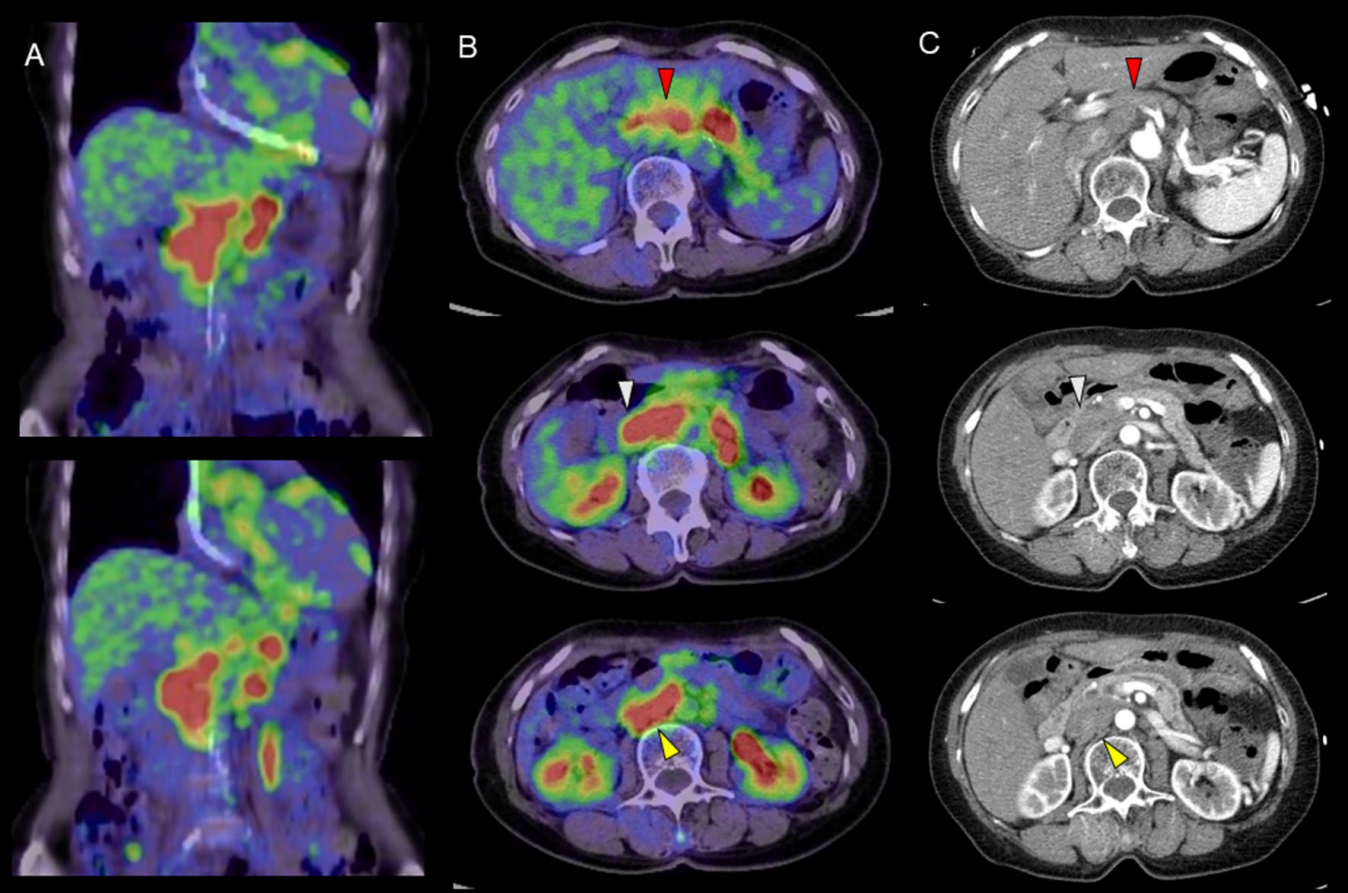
ACoronal images of fused PET/CT image, B axial images of fused PET/CT, C axial images of contrast CT. Intense FDG uptake was observed in multiple swollen lymph nodes: the periportal lymph node (upper row, red arrowheads), anterior pancreaticoduodenal lymph node (middle row, white arrowheads), and posterior pancreaticoduodenal lymph node (lower row, yellow arrowheads), along with uptake in the celiac lymph node
